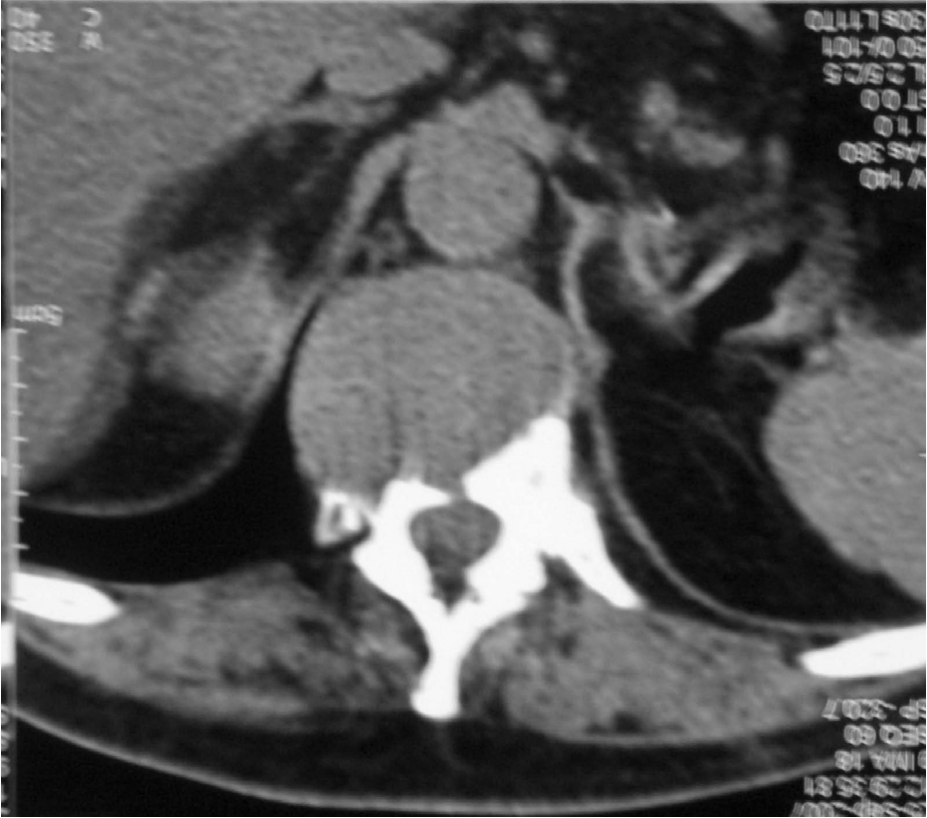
Chest CT of lesion. Chest CT showing osteolytic lesion of D11 eroding anterior cortex and superior endplate and involving superior vertebral body.
